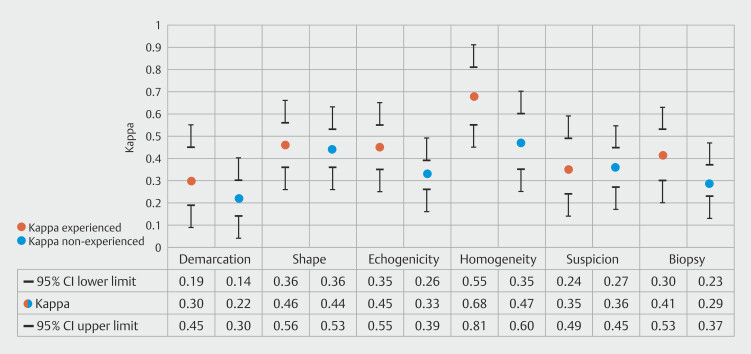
Kappa values per LN characteristic of experienced vs non-experienced endosonographers. CI, confidence interval.
